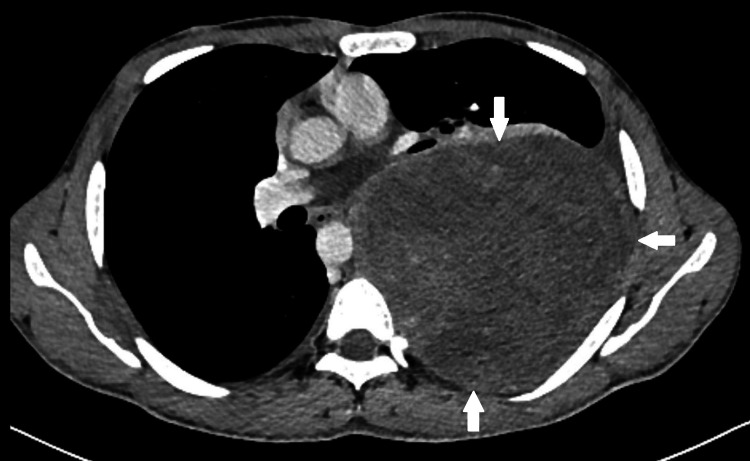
Axial section of contrast-enhanced CT chest shows a large mildly enhancing mass lesion along posterior pleura (white arrows), which is causing mass effect over the left lung parenchyma
